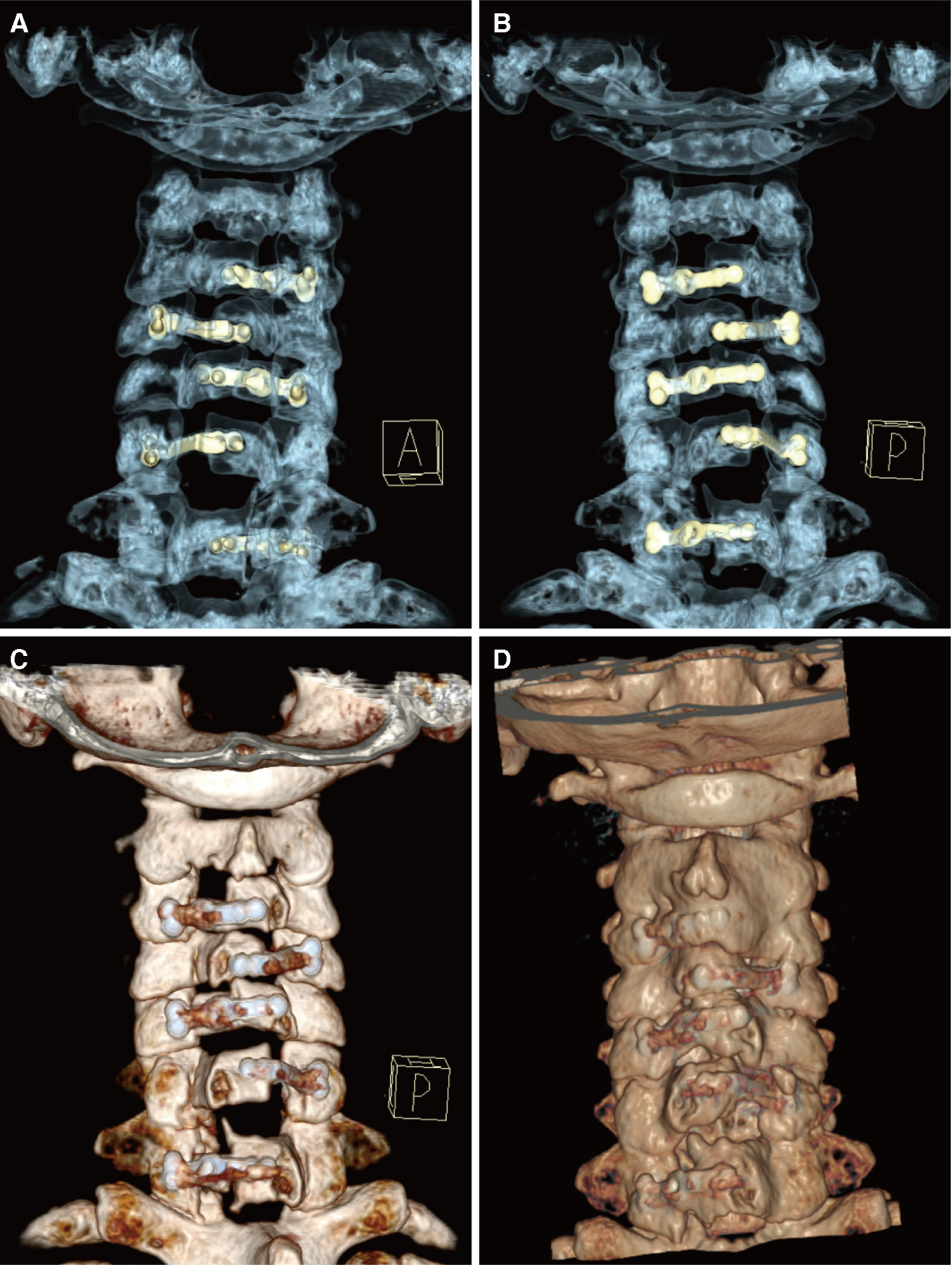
(A,B) Three-dimensional reconstructions of CT images (A: anterior view, B: posterior view), to allow clear visualization of the operating feature of the internal fixation plates during this novel technique. (C,D) Posterior views of the reconstructed cervical spine structure (C: 1 week postoperatively, D: 5 years postoperatively), showing the fixation and integration of the implanted plate with the posterior lamina. CT, computed tomography.
